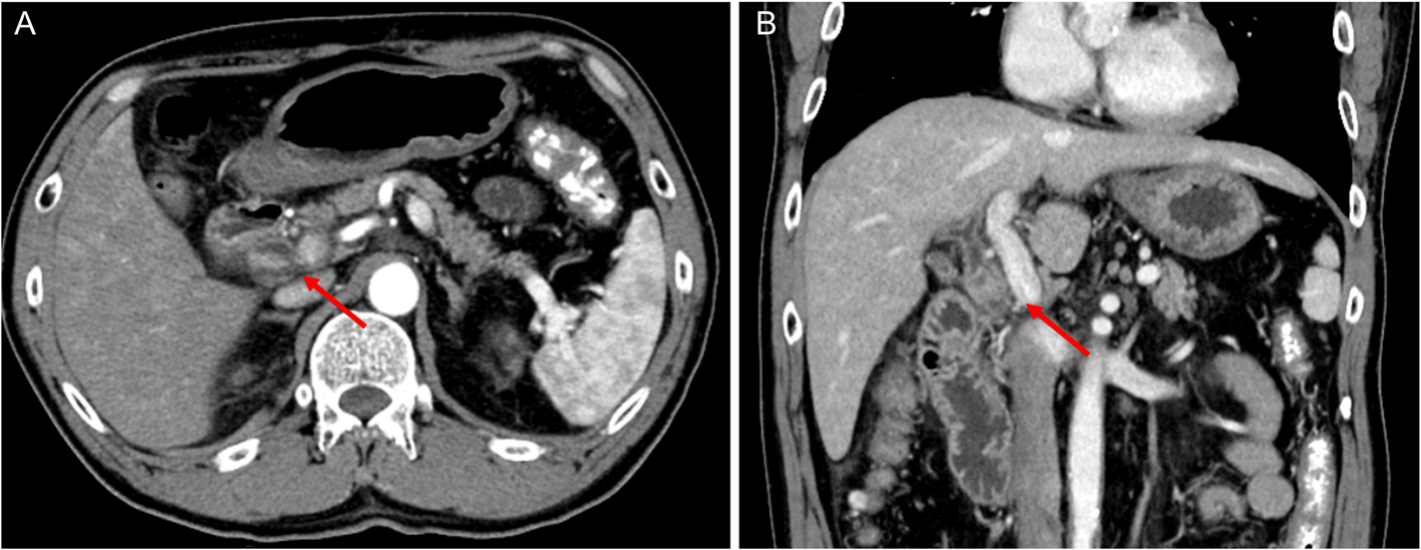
Enhanced CT scan images after EST. Enhanced CT scan showing improved dilation of the CBD and enhanced wall thickness (arrows) of the bile duct measuring 25 × 10 mm at the union of the cystic and common hepatic ducts (a, b)
